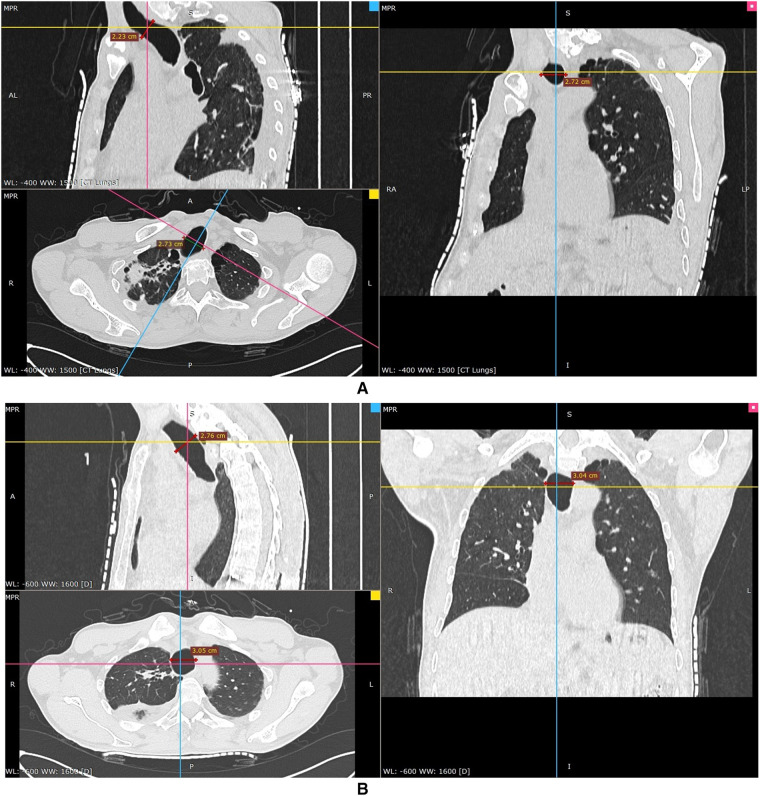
CT images of the lung demonstrating an enlarged trachea on the level of 2 cm above the aortic arch. The axial image (down-left) and coronal image (right) showed that the diameter of the trachea was 27.2 mm. The sagittal diameter (up-left) of the trachea was 22.3 mm (A). CT images of the lung on the level of the aortic arch, where the diameter of the trachea was the longest. The transverse diameter on the down-left axial image was 30.5 mm. The diameter on the right coronal image was 30.4 mm. Also, the sagittal image (up-left) demonstrated that the diameter of the trachea was 27.6 mm (B).
